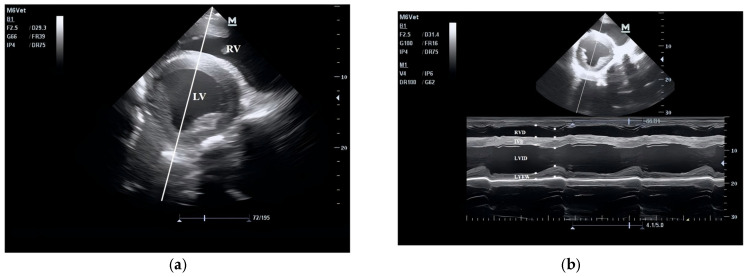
B-mode (a) and M-mode (b) echocardiogram of the right parasternal short-axis view.
